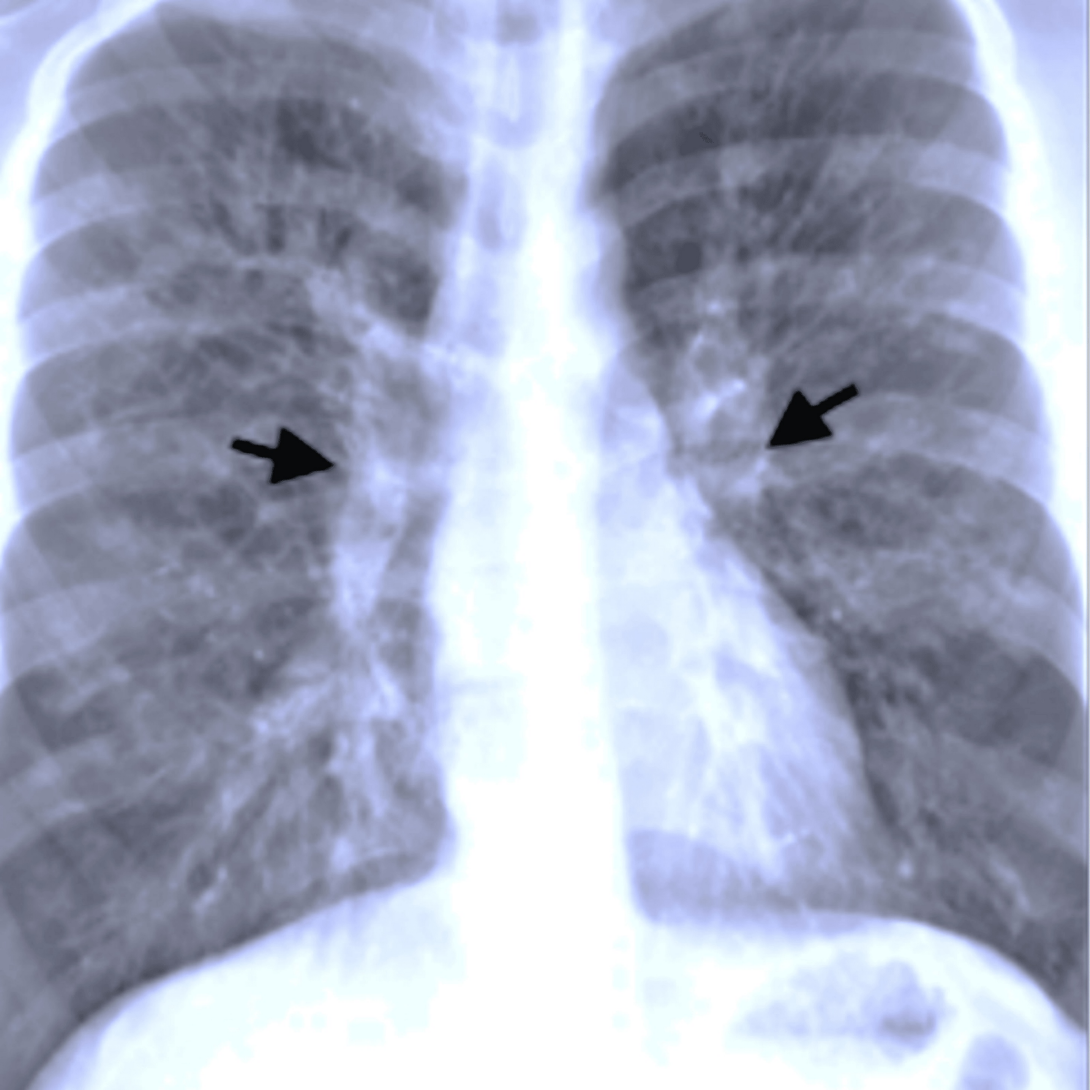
Preoperative chest X-ray revealing pulmonary plethora in the patient's native lung
